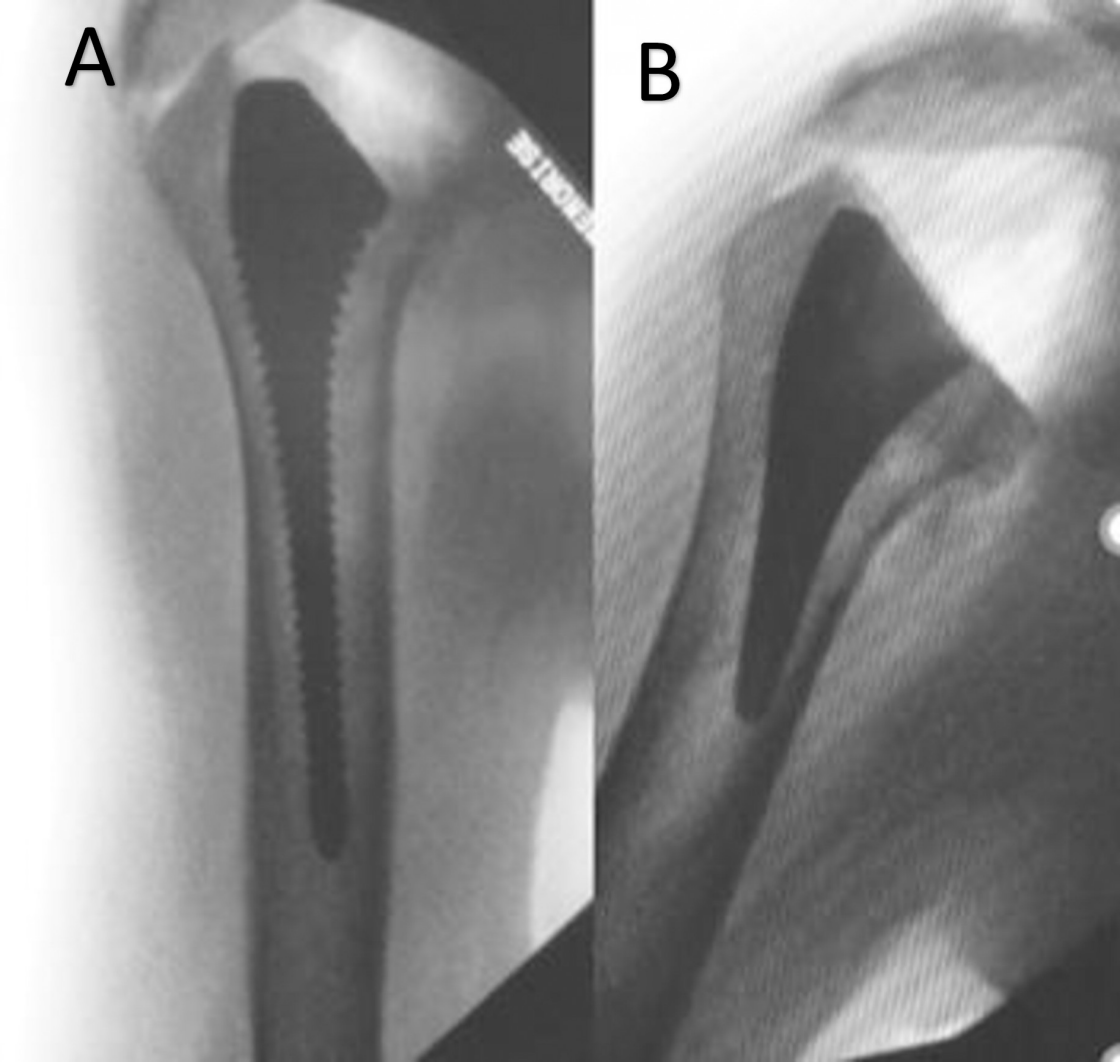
True anteroposterior humeral radiographs showing valgus positioned implants (size 7) (A) trial (alpha angle 6,8°) (B) short-stem (alpha angle 8,2°) in the same subject.
